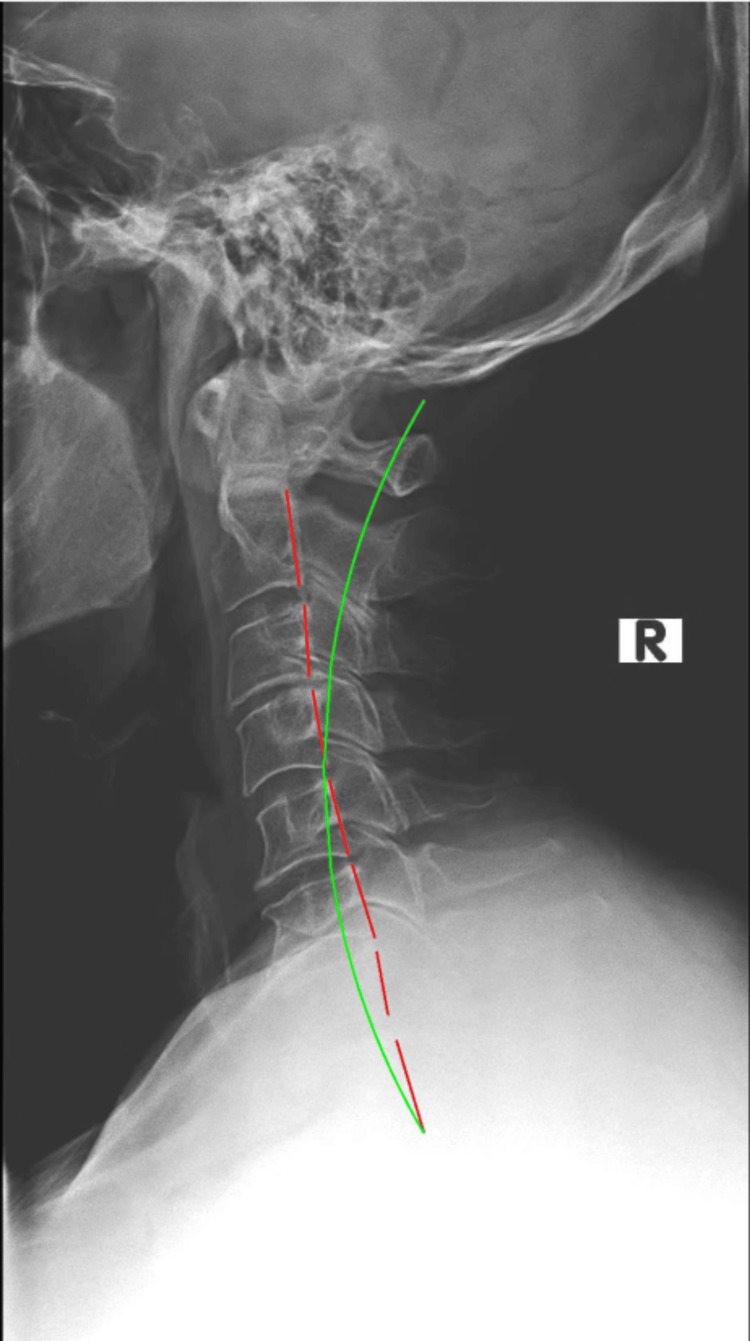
Initial lateral cervical radiograph The red dashed red line demonstrates the Harrison Posterior Tangent Method of mensuration. The green line represents ideal cervical lordosis.
